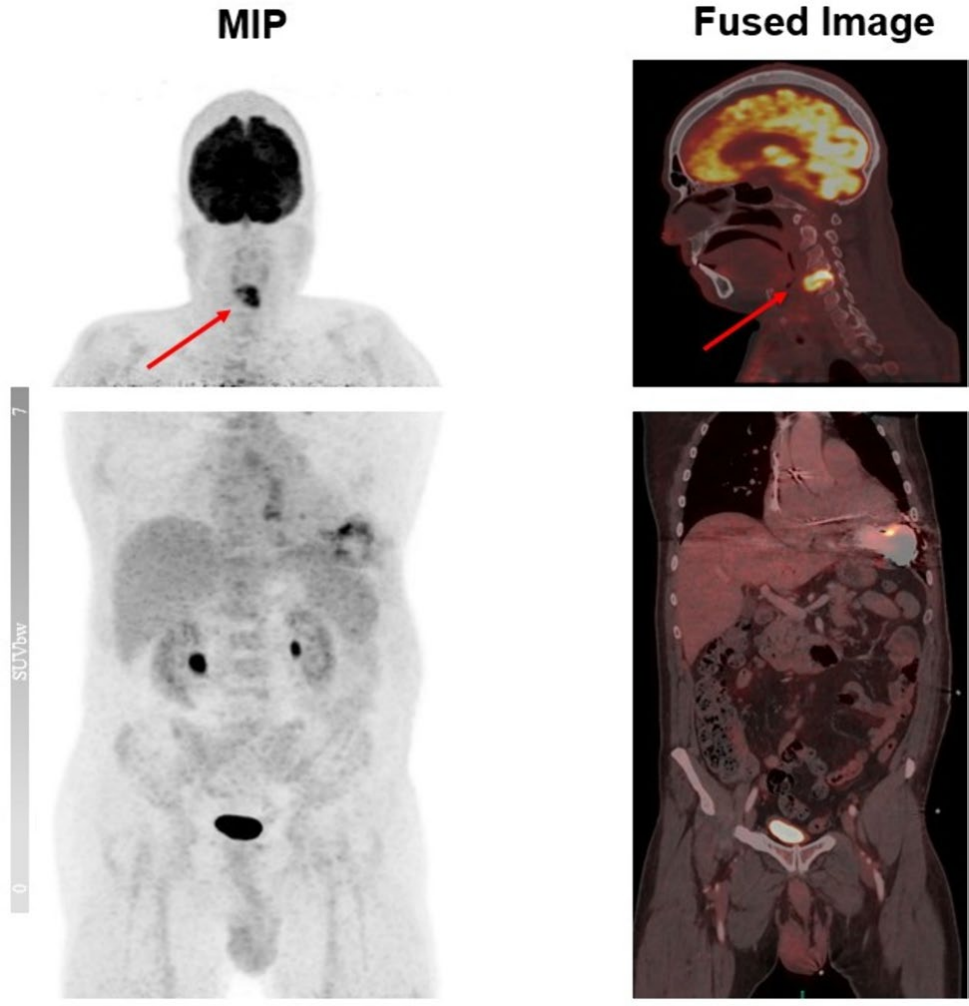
67-year-old male patient undergoing [^18^F]FDG PET scan due to suspected VAD-specific infection, which reveals an acute spondylodiscitis in the cervical spine as a typical case of non-VAD-related infection. Around LVAD is only a moderate [^18^F]FDG uptake to be observed in the pump housing as a means of attenuation artifacts
